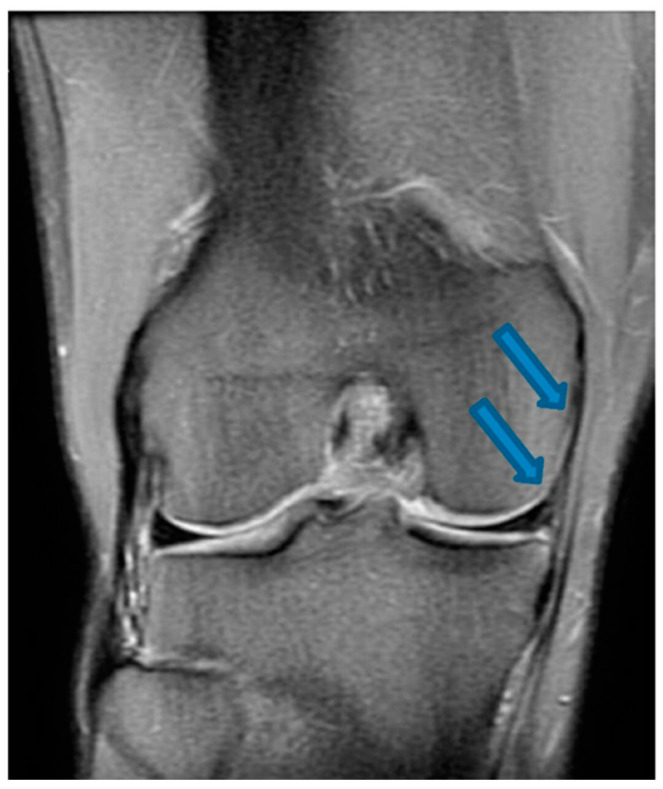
Coronal T2-weighted MRI of the knee showing complete MCL and medial meniscus healing indicated by blue arrows (24 December 2021).
